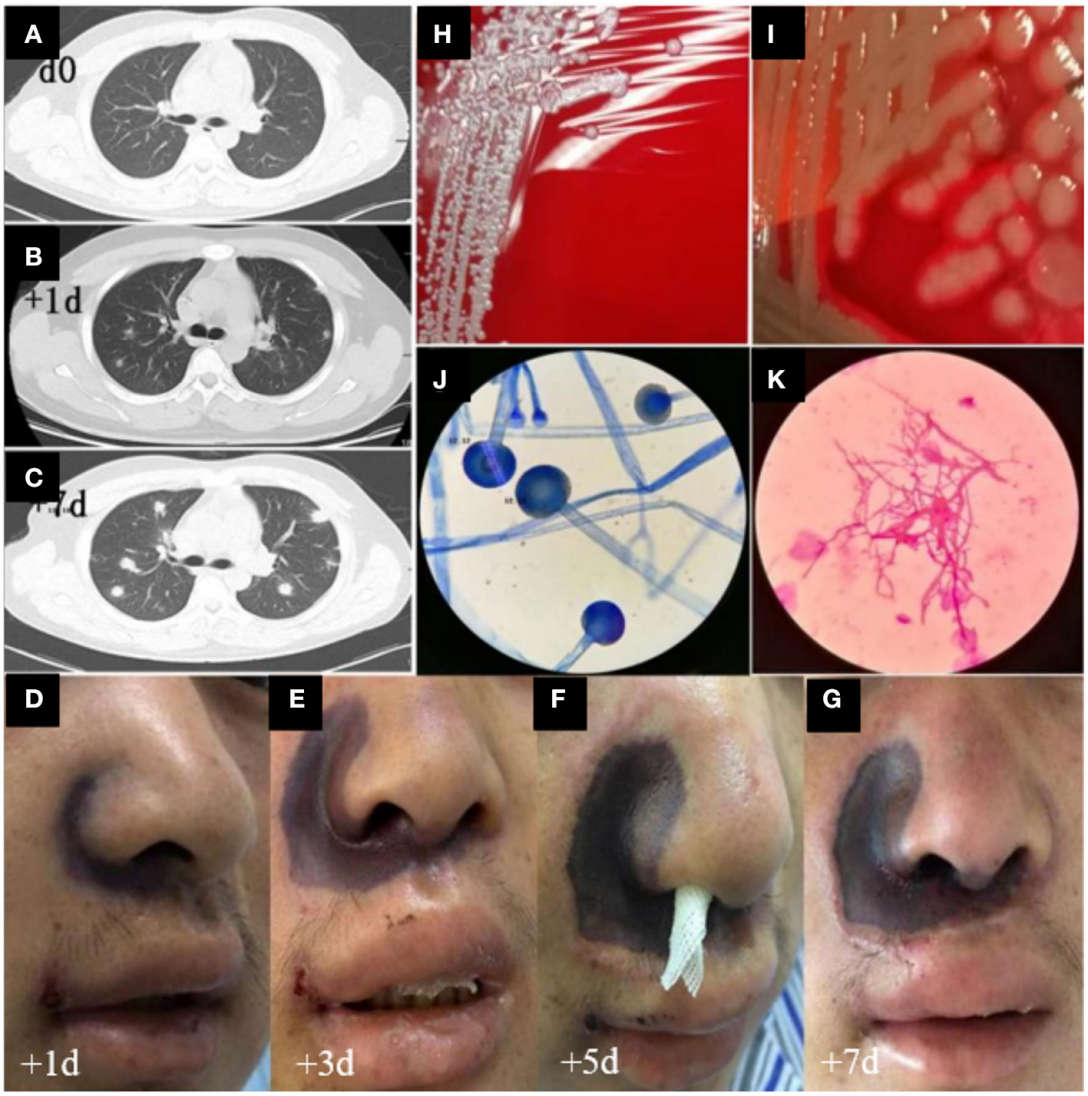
CT images, advancement of skin damage, and morphologic features of microbiological cultures in this case. (A–C) Day 0, day 1, and day 7 of the chest CT images, respectively. (D–G) Day 1, 3, 5, and 7 images of the nasal skin, respectively. (H,I) Bacterial cultures of *Staphylococcus haemolyticus* and MDR *Pseudomonas aeruginosa*, respectively. (J,K) The morphologic features of Mucor.
